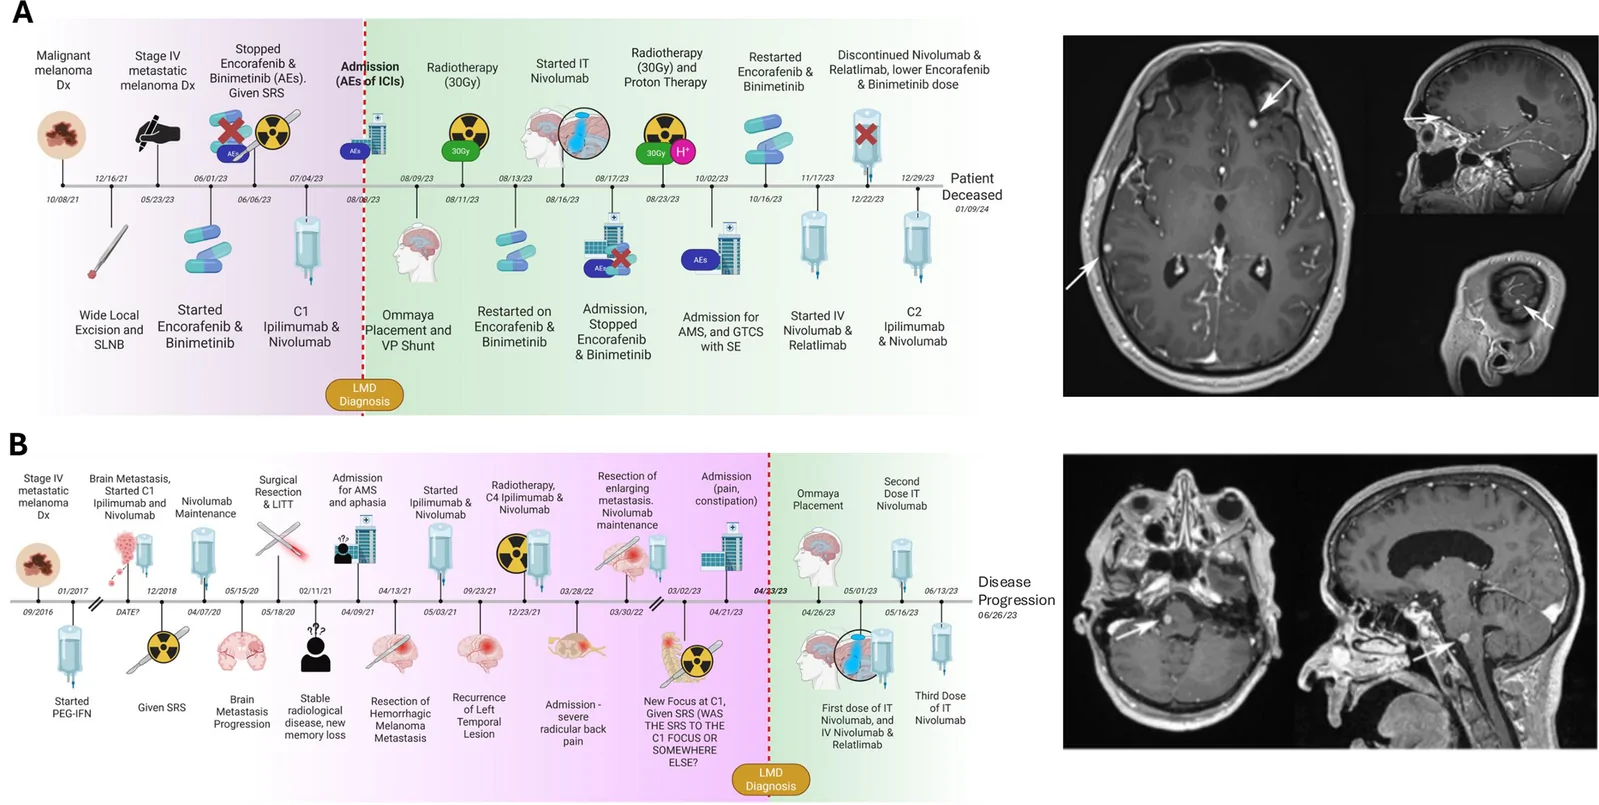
Detailed treatment timeline and representative magnetic resonance imaging (MRI) of Patient 1 (A) and Patient 2 (B)
